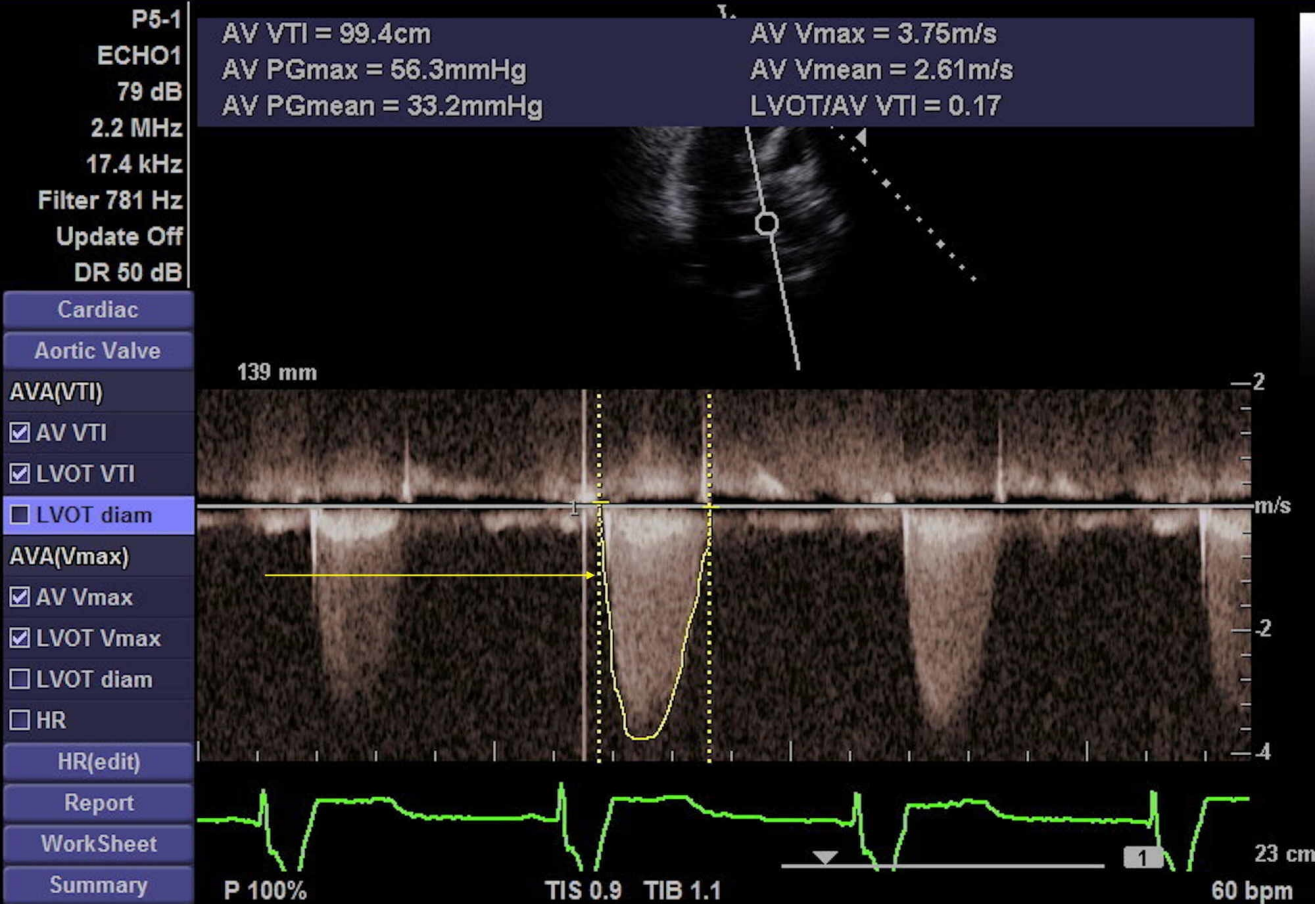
Transthoracic Echocardiogram (TTE) TTE shows moderate to severe aortic stenosis
